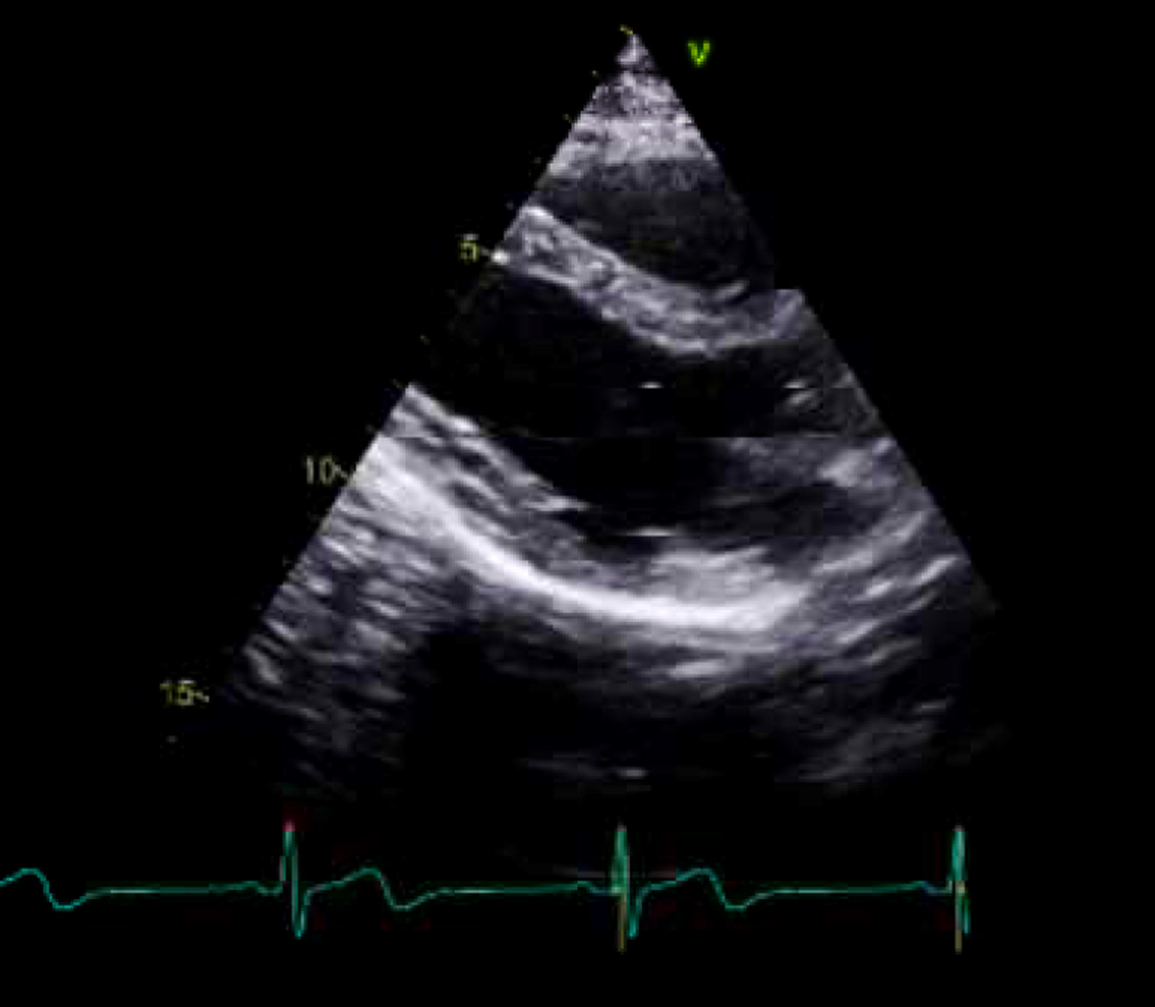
Normal echocardiogram (Echo).
